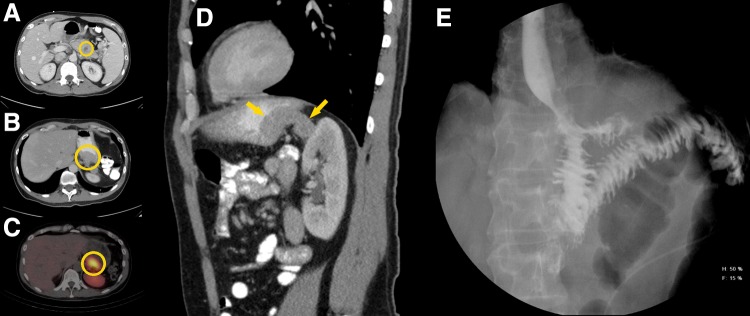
(A) Initial abdominal CT scan demonstrating pancreatic body lesion (yellow circle). (B) Abdominal CT scan 1 year after distal pancreatectomy and splenectomy with gastric mass visible (yellow circle). (C) Octreotide scan with enhancement of the posterior gastric wall (yellow circle). (D) Sagittal cut of abdominal CT scan showing gastric mass extending posteriorly (yellow arrows) toward the left adrenal gland. (E) Postoperative gastrographin swallow showing anatomical reconstruction through esophagojejunostomy.
